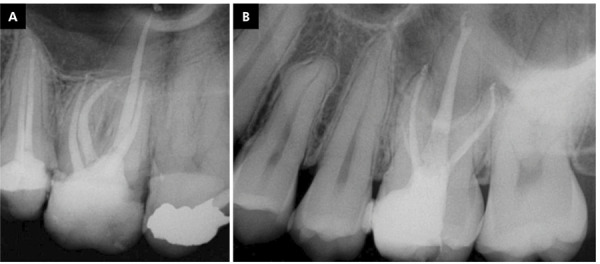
Radiograph showing the root canal filling in a case considered as negotiable (A) and a case considered as non-negotiable (B).
